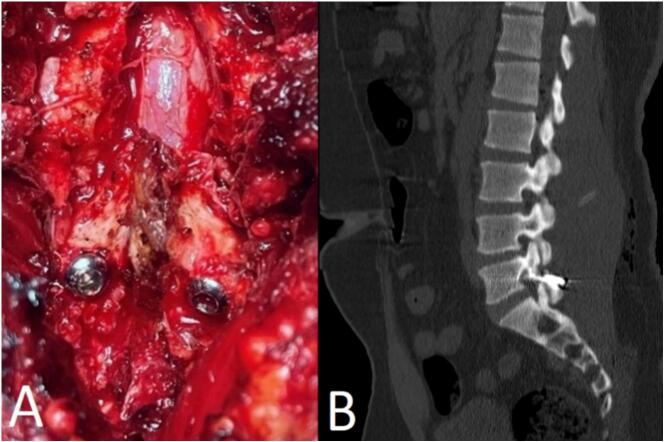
Intraoperative and postoperative imaging. Intraoperative photograph showing the successful placement of bilateral cortical screws across the pars defects at the L5 level using Buck's technique, with autologous bone graft packed around the screws (A). Postoperative sagittal CT scan demonstrating proper screw placement across the L5 pars defects with intact vertebral alignment and no evidence of spondylolisthesis or residual instability (B).
